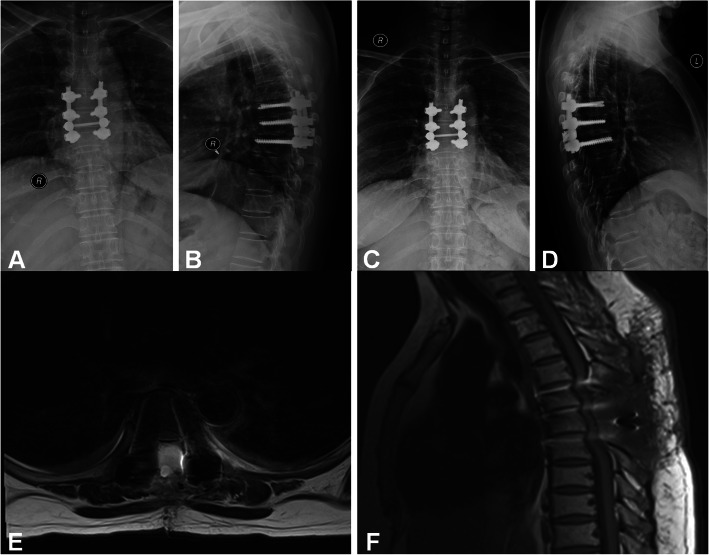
Patient with intraspinal tumour treated by laminectomy with pedicle screw fixation. a and b At 6 months following the surgery, X-ray examination indicated no fixation transposition or fracture, lumbar instability or kyphosis. c and d At 12 months following the surgery, X-ray examination indicated no fixation transposition or fracture, lumbar instability or kyphosis. e and f Twelve months following the surgery, MRI showed that the vertebral canal shape was irregular, and the dural sac was partially compressed
